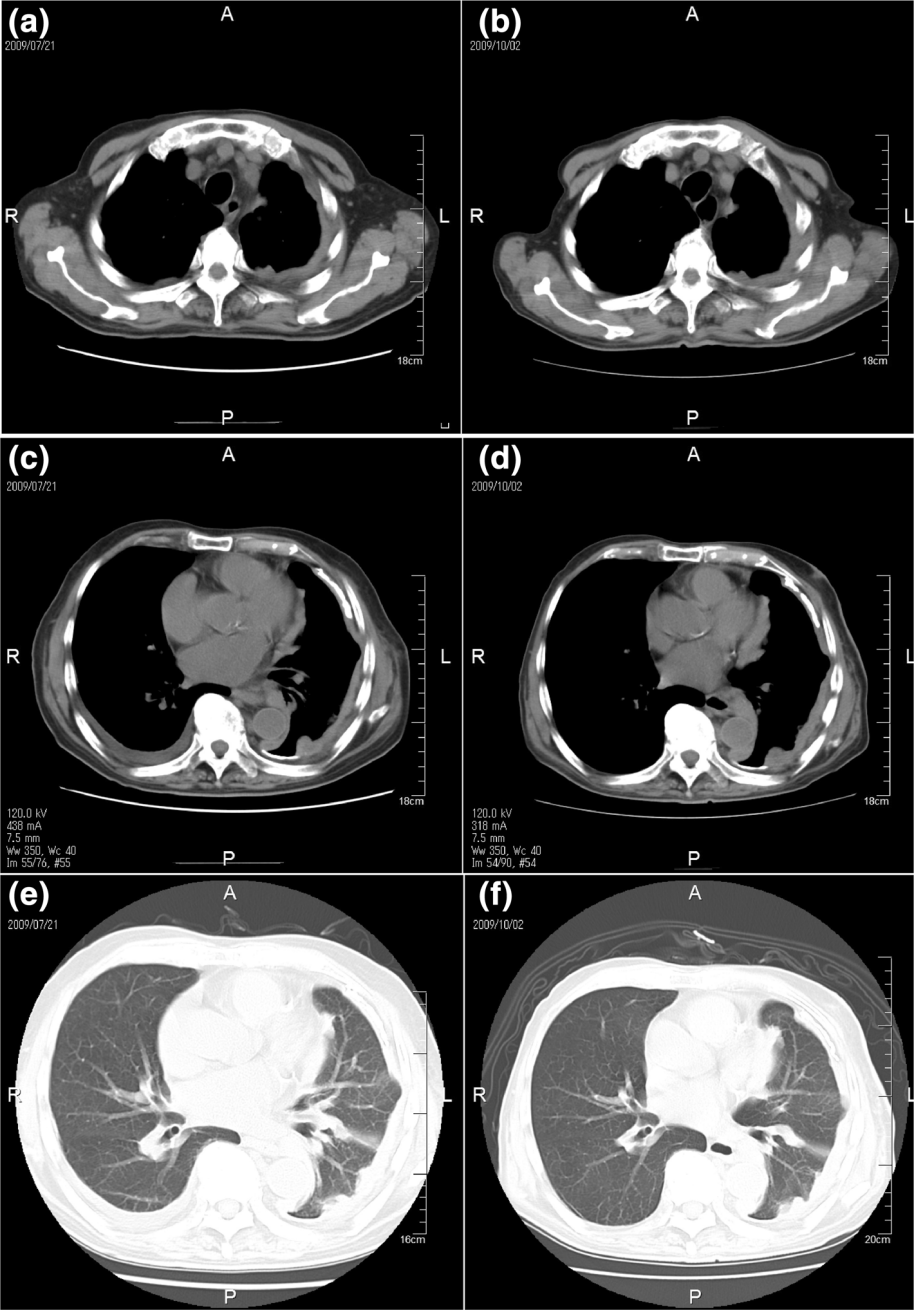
Chest computed tomography of DMM lesions (Case 3) before (a), (c), (e) and after cisplatin and pemetrexed chemotherapy (b), (d), (f)
